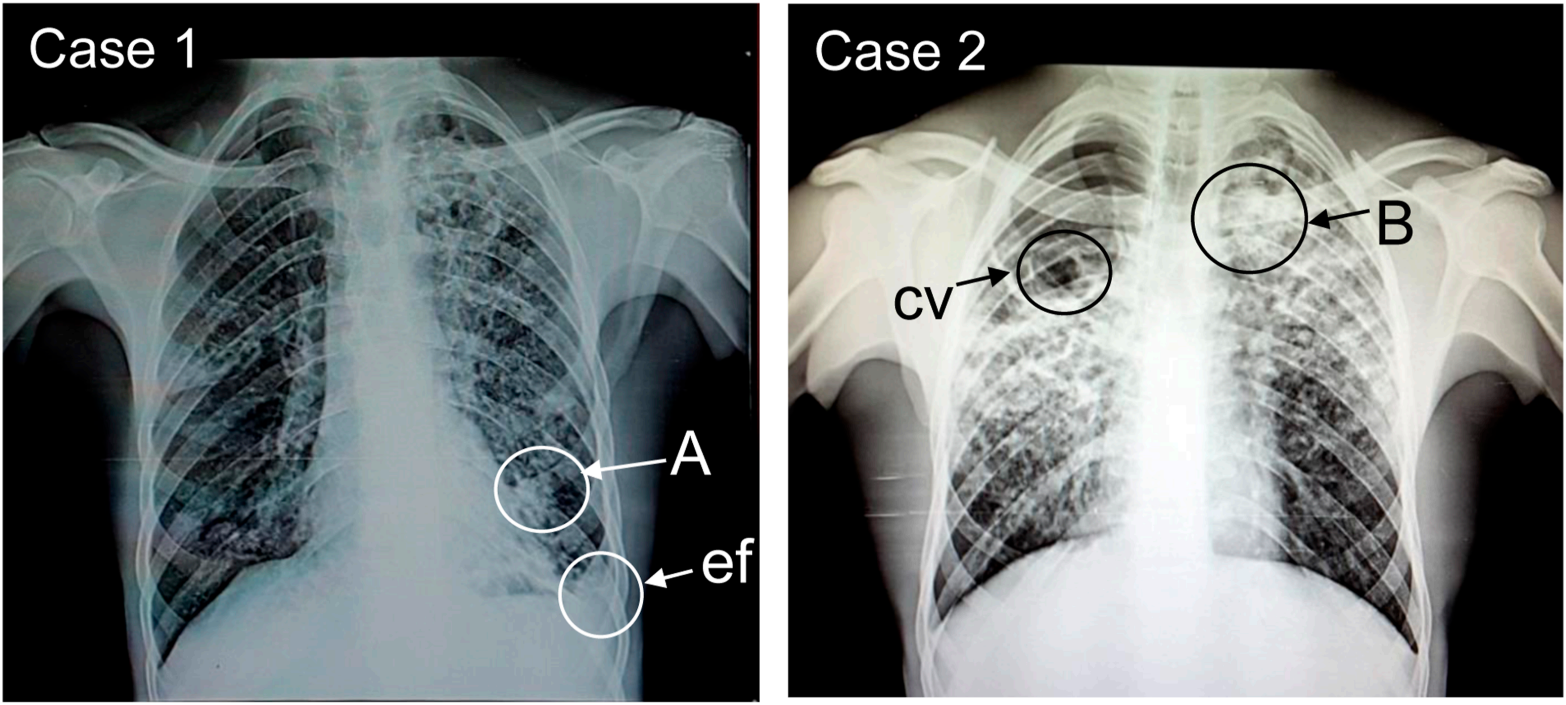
Chest X-rays from two mineworkers, with interpretation according to the ILO International Classification of Radiographs of Pneumoconioses Revised, 2022 edition. Case 1: Radiograph quality Grade 2 (right rotation and inadequate penetration), showing small round opacities, shape and size (q/q) and profusion (2/2), localised in the right middle and lower zones, as well as in the left upper, middle and lower zones. A large opacity (A) next to the left heart border and pleural abnormality: left costophrenic angle obliteration by pleural effusion (ef). In the absence of TB, these radiological features are compatible with silicosis. Case 2: Radiograph quality Grade 2 (left rotation and inadequate penetration) showing diffuse small round opacities, size and shape (r/q), profusion (3/2), localised in the right, middle and lower zones, as well as the left upper, middle and lower zones, and large opacities (B) in the left upper zone and background ground glass opacities; cavity (cv) in right upper zone. These radiological features are suggestive of silico-TB.
